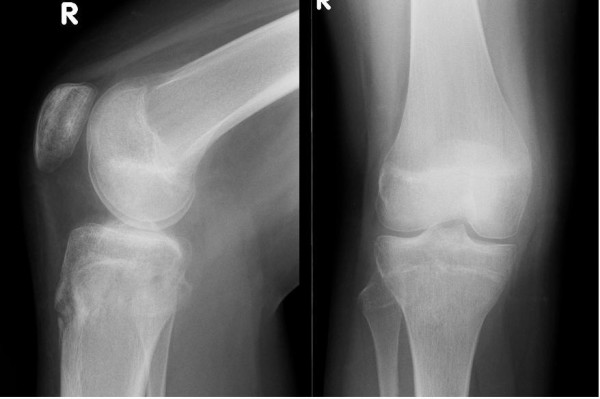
Six months post-operative radiographs.
